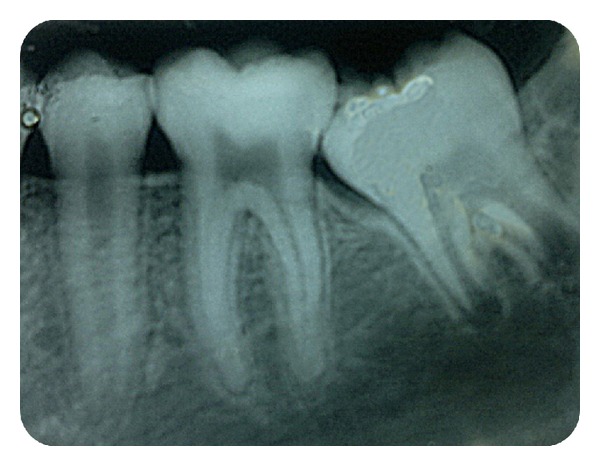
Intraoral periapical radiograph showing permanent mandibular second molar locked beneath first permanent molar.
